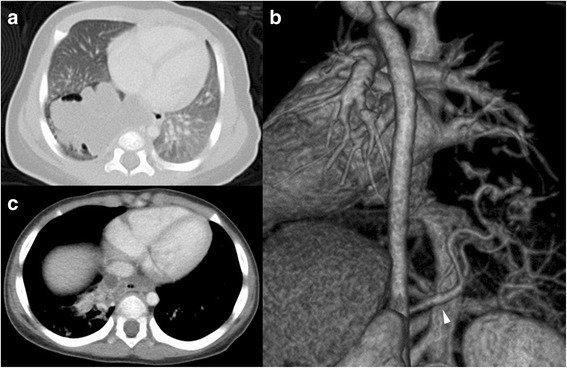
A chest computed tomography scan obtained from Patient 1 at 29 days of life. a Diffuse miliary nodules and low-density masses are demonstrated in the right lower lung and posterior mediastinum. b An aberrant arterial supply arising from the proximal celiac axis is indicated (arrowhead). c The masses appear to be connected to each other through the pulmonary ligament
